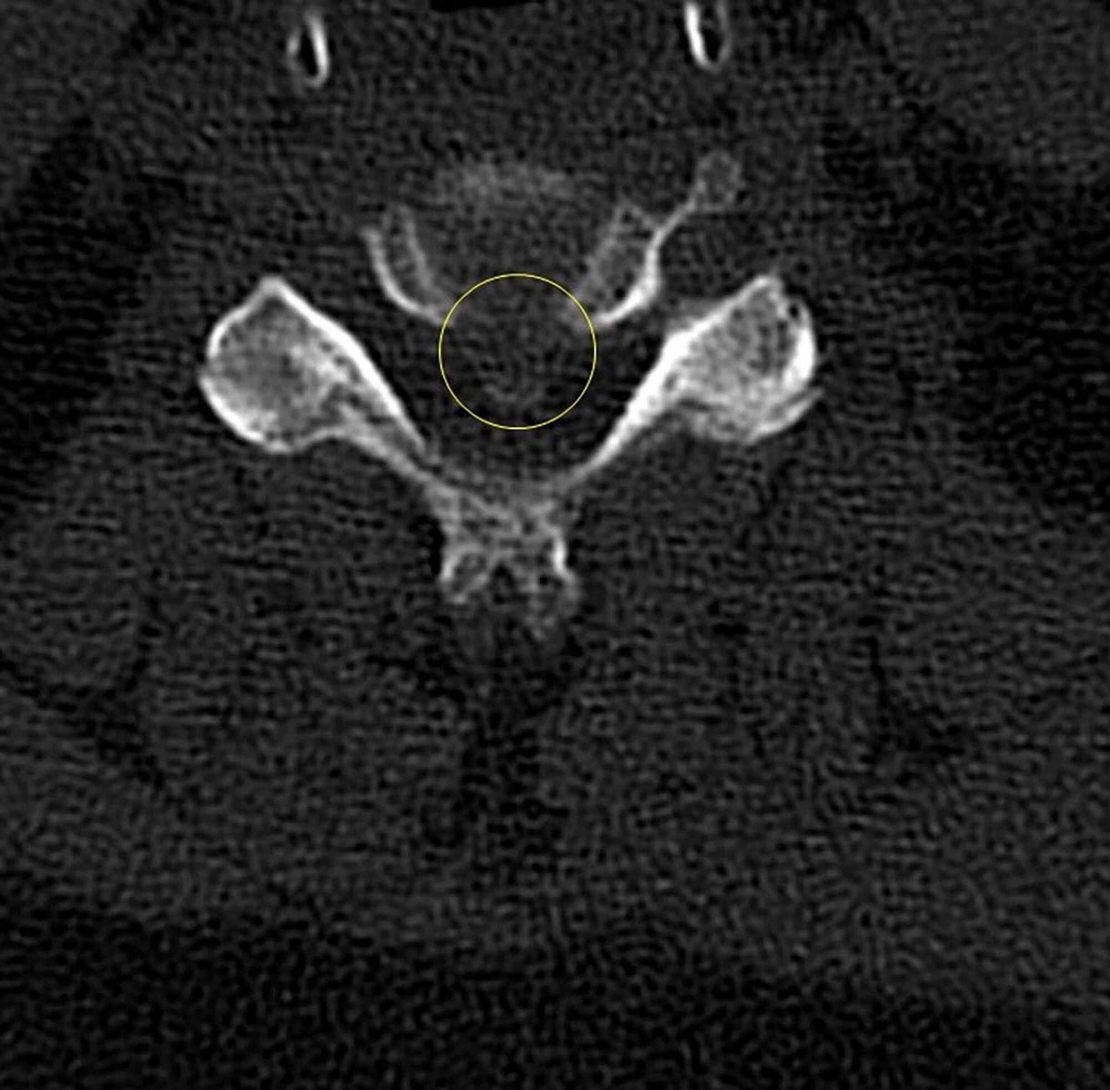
Axial computed tomography scan cut at the level of C5 showcasing severe ossification of the posterior longitudinal ligament (yellow circle) with significant protrusion into the spinal canal.
